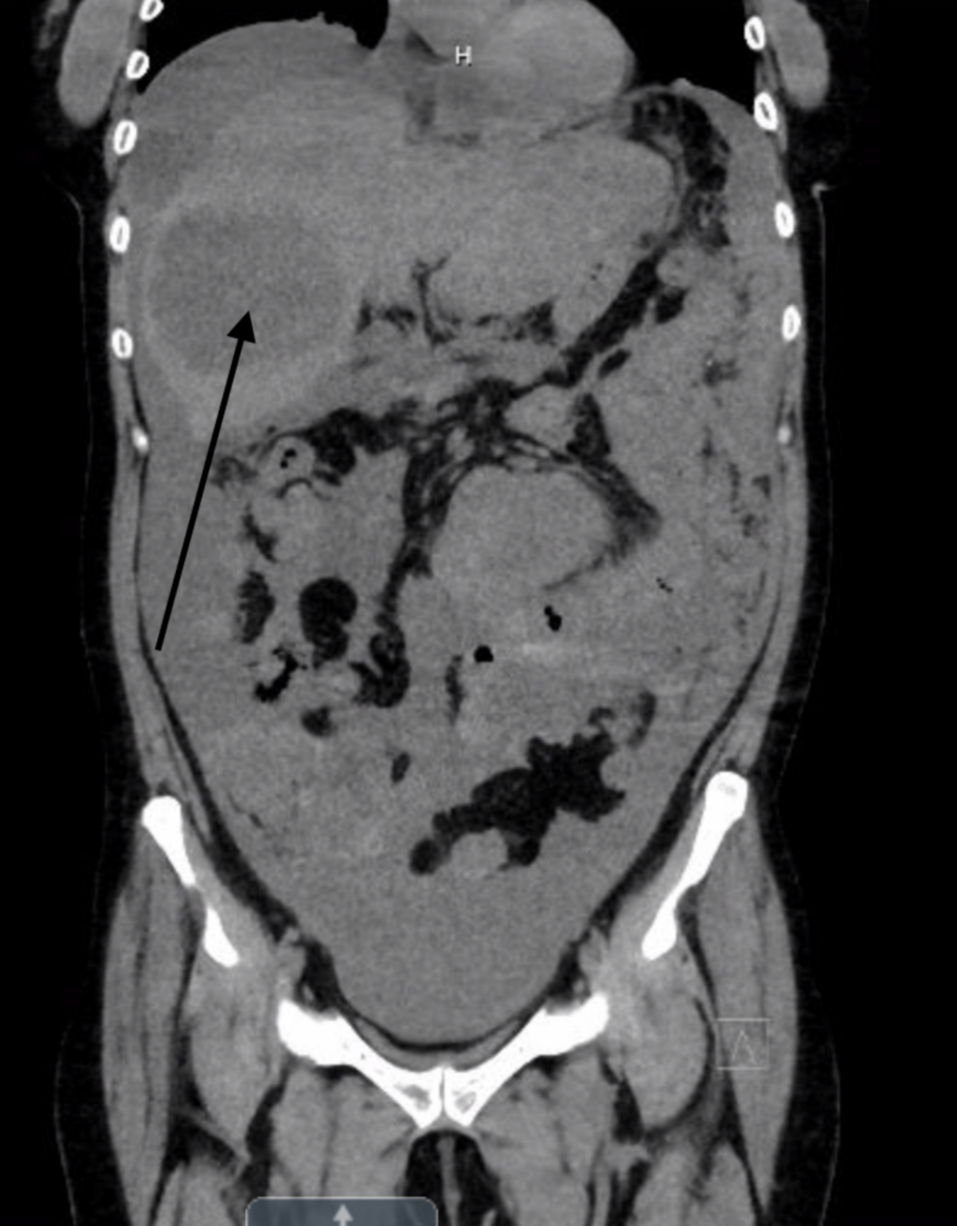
Coronal view of CT chest and abdomen Coronal view of CT chest and abdomen showing a large right hepatic lobe mass (black arrow) measuring 8.7 cm x 8.7 cm and fluid in the right upper quadrant.
